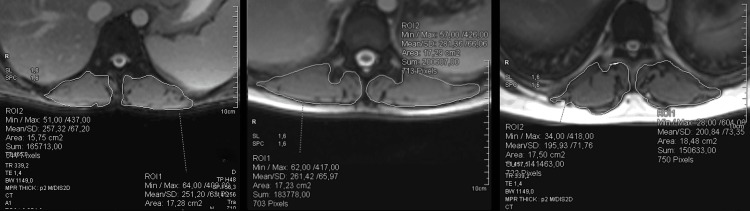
Manual segmentation and region of interest area calculation of the dorsal muscle compartment at the level of the 12th thoracic vertebra (detail, MRI) MRI: magnetic resonance imaging
